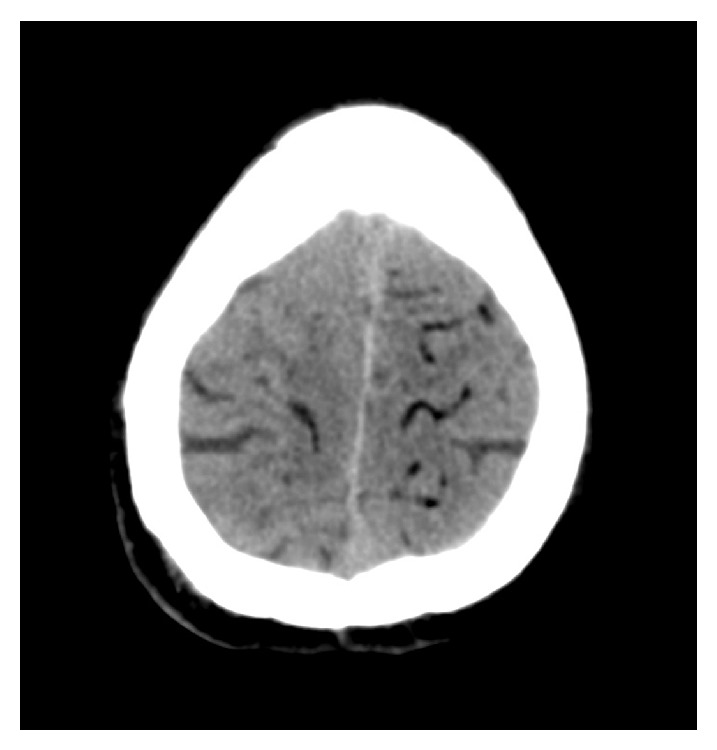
CT image of the brain after lung biopsy with signs of cerebral air embolism, typically visible as subcortical serpentiform formations with negative Hounsfield units.
